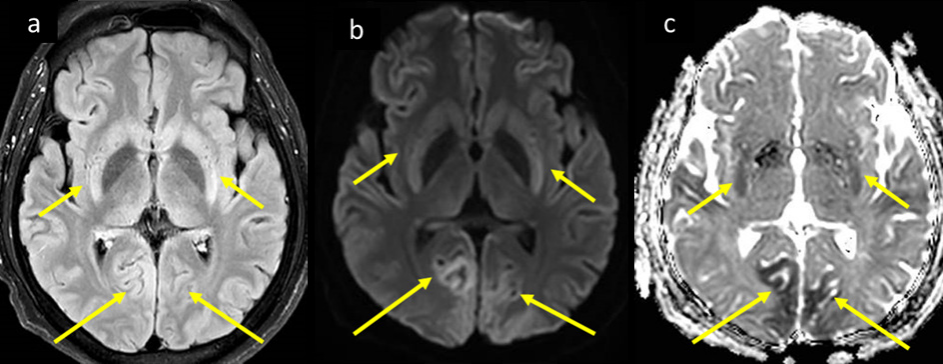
A 49-year-old COVID-19 positive male progressed to ARDS, and developed cardiac arrest for short period during endotracheal intubation. MRI brain axial images at the level of basal ganglia, (a) *T*_2_-FLAIR, (b) DWI b1000, and (c) ADC map, shows bilateral cerebral gyral and basal ganglia swelling and bright signal on *T*_2_-FLAIR, corresponding diffusion restriction with high signal on DWI signal and low signal on ADC map, consistent with hypoxic ischemic encephalopathy (long and short arrows respectively in a–c). ADC, apparent diffusion coefficient; ARDS, acute respiratory distress syndrome; DWI, diffusion-weightedimage; FLAIR, fluid attenuated inversion recovery; ICU, intensive care unit.
